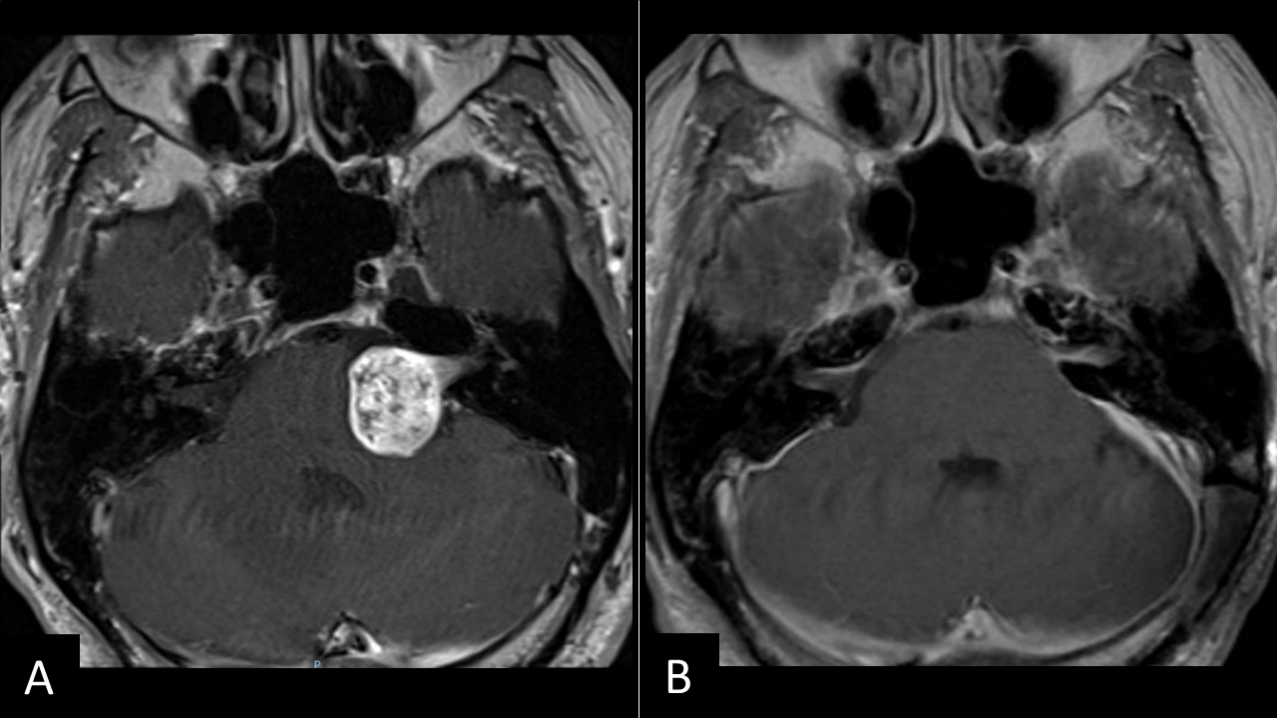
Vestibular Schwannoma Before and After Resection T1-weighted magnetic resonance imaging (MRI) demonstrating the left 2.7 cm vestibular schwannoma (A) before and (B) nine months after surgical resection and reconstruction of the internal auditory canal (IAC) bone with hydroxylapatite.
